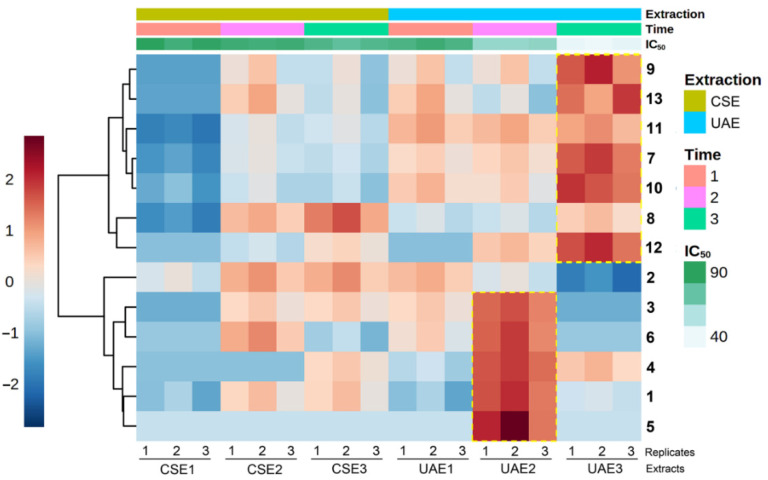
Distribution of the relative abundance of the quinolizidine alkaloids (1–12) and amide 13 detected in extracts of *L. mirabilis* under different extraction conditions. CSE1-3: conventional solvent extraction for 2, 4, and 6 h, respectively; UAE1-3: ultrasound-assisted extraction for 0.5, 1, and 2 h, respectively. The heat map is organized by columns for each factor, i.e., the extraction type (CSE and UAE), extraction time (1, 2, and 3), and mycelial growth inhibition expressed by green shades (dark green: IC_50_ = 90 µg/mL; light green: IC_50_ = 40 µg/mL). Cells colored by autoscaled relative abundance of each alkaloid (dark red: high abundance; and dark blue: low abundance). The heat map is also organized by rows according to the Ward clustering algorithm (Euclidean distance).
